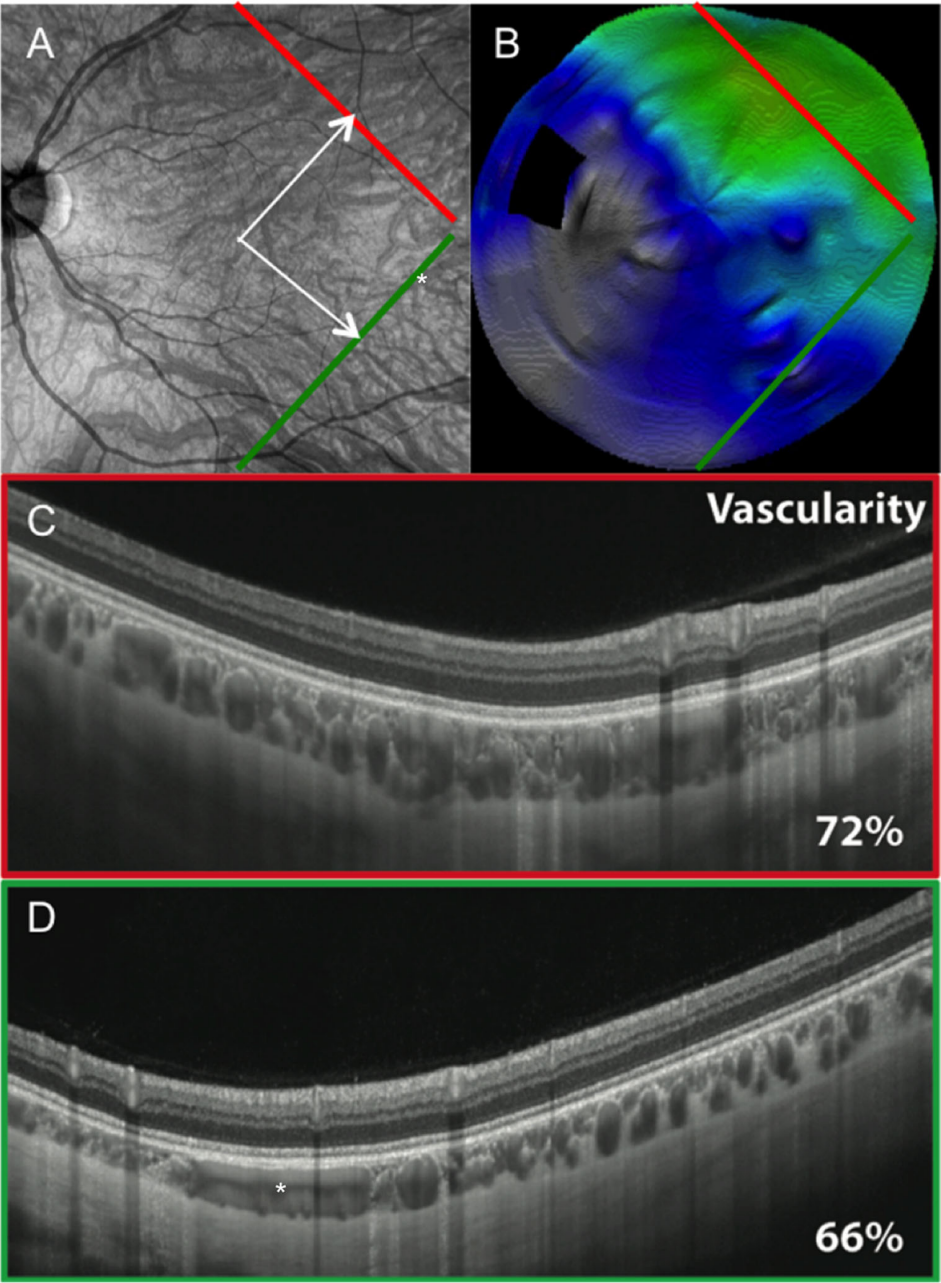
Vascularity index measurement. The vascularity index (VI) corresponds to the surface of the vessel lumen divided by the overall surface of the choroid. Two B-scans passing perpendicularly to the direction of the most choroidal veins in asymmetrical patterns were acquired superiorly and inferiorly with respect to the horizontal axis, at equidistance from the fovea, in the temporal region. The figure shows (A) a 9 × 9-mm en face OCT-A image and (B) the corresponding 12-mm radial CT map of a medium-thin asymmetrical choroid. The area of vessel lumen (dark on OCT B-scans) was determined based on a semiquantitative method. (C) B-scan used for VI measurement in the superotemporal region. (D) B-scan used for VI measurement in the inferotemporal region. In this example, the VIs were 72% and 66%, respectively, in the thickest and thinnest area of the CT map. In this image, one vessel is tangential to the B-scan, visible on the en face image (asterisk).
